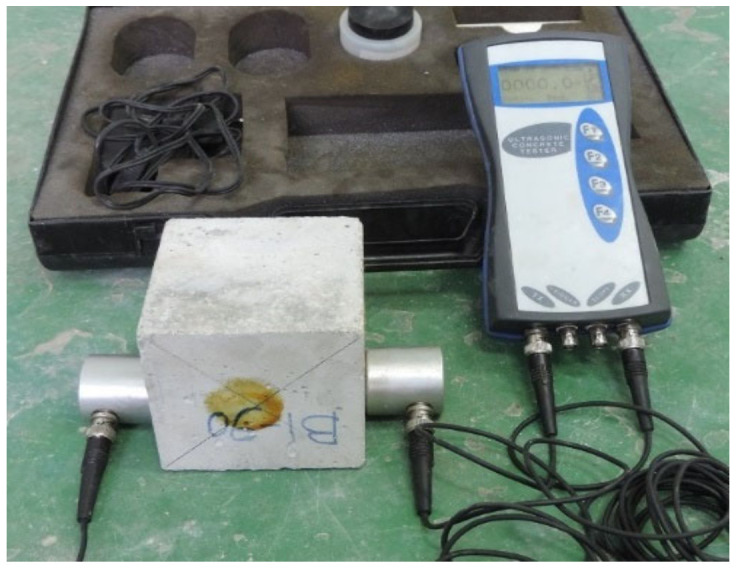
The ultrasonic pulse velocity test device.
